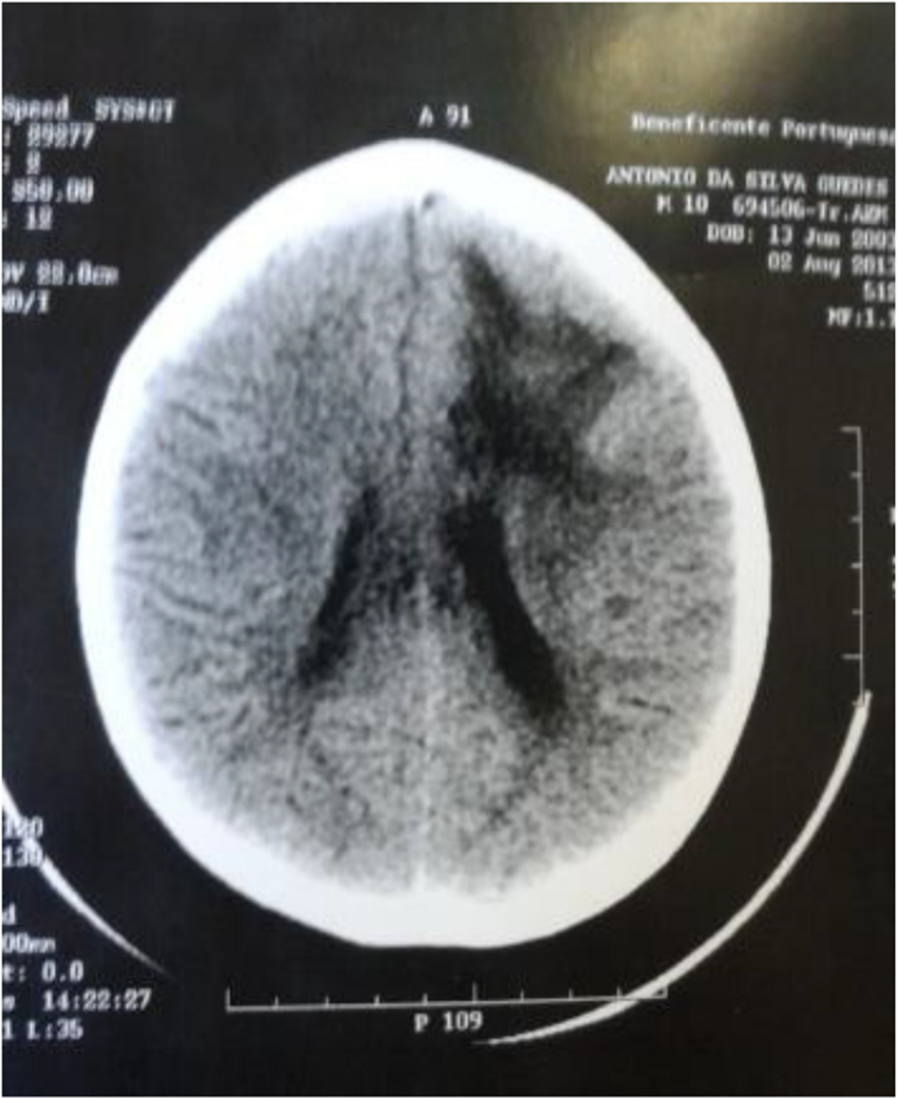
Cranial computed tomography showing intracerebral hemorrhage in the right frontal area
